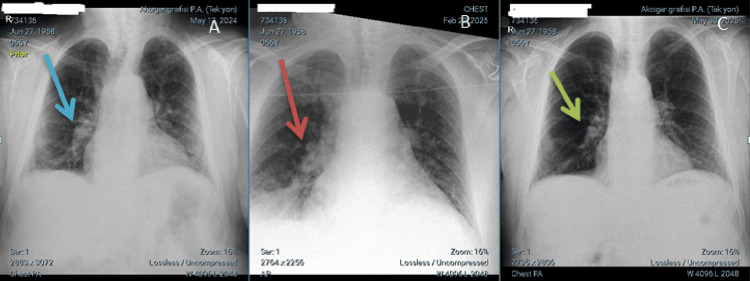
Chest radiographs from A to C obtained in outpatient clinic, consultation, and discharge, respectively. (A) The blue arrow indicates a dilated pulmonary artery in the patient with COPD. The cardiothoracic ratio is slightly increased, and the heart shadow is slightly enlarged. (B) The cardiothoracic ratio and cardiac shadows are enlarged. The red arrow indicates the dilated right main pulmonary artery and pulmonary edema. (C) The cardiothoracic ratio decreased, and the dimensions of the heart shadow returned to baseline. The green arrow shows a shrunken pulmonary artery. P.A.: posterior anterior; COPD: chronic obstructive pulmonary disease
